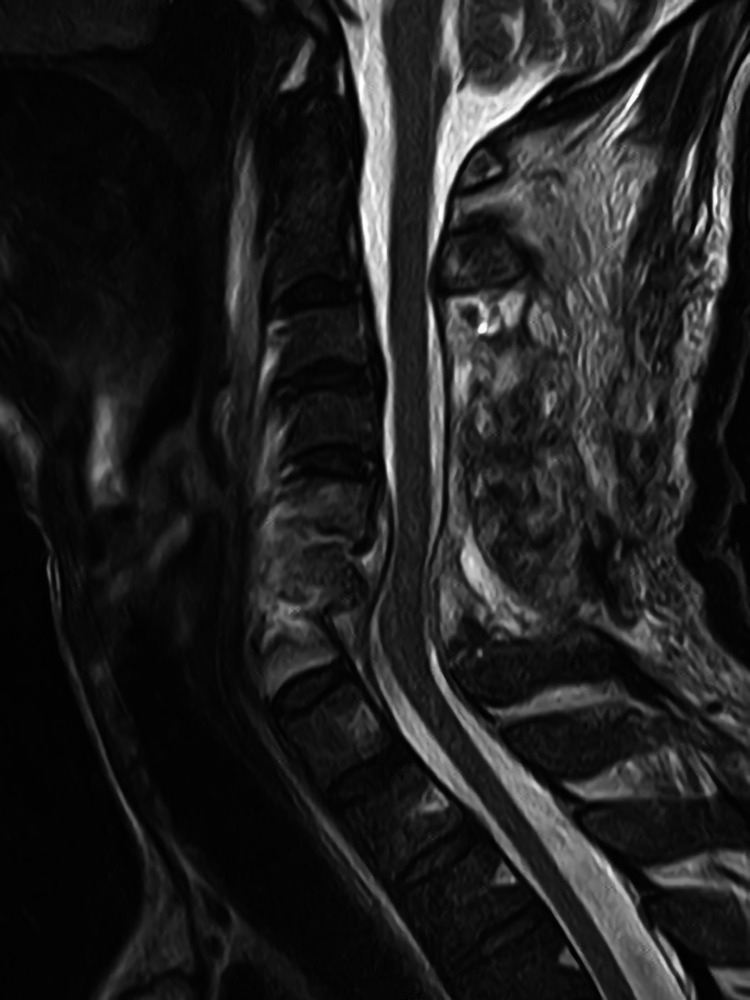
Cervical plain MRI after the first surgery Sagittal view of cervical T2-weighted MRI after laminectomy. Sufficient decompression of the spinal cord was achieved.
